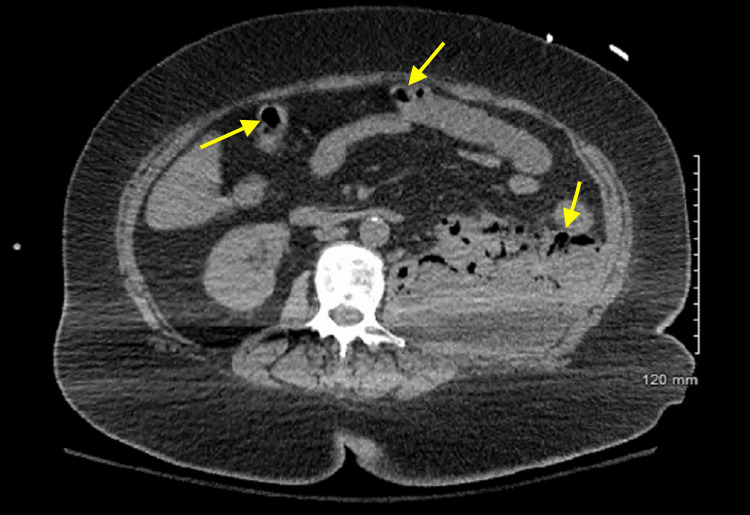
Computed tomography of the chest demonstrating retroperitoneal air within various parts of the colon (yellow arrows), which is concerning for necrotizing fasciitis
